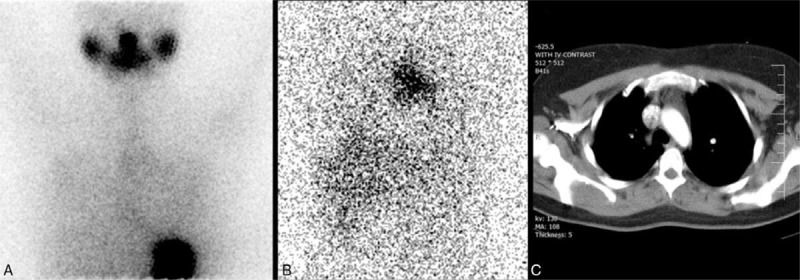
(A) Iodine-131 WBS 48 hours after second treatment reveals faint uptake in the anterior mediastinum. (B) ^131^I WBS 14 days later reveals intense uptake in the same region. (C) Soft tissue attenuation mass(thymus) reveals in the upper mediastinum on CT scan.
